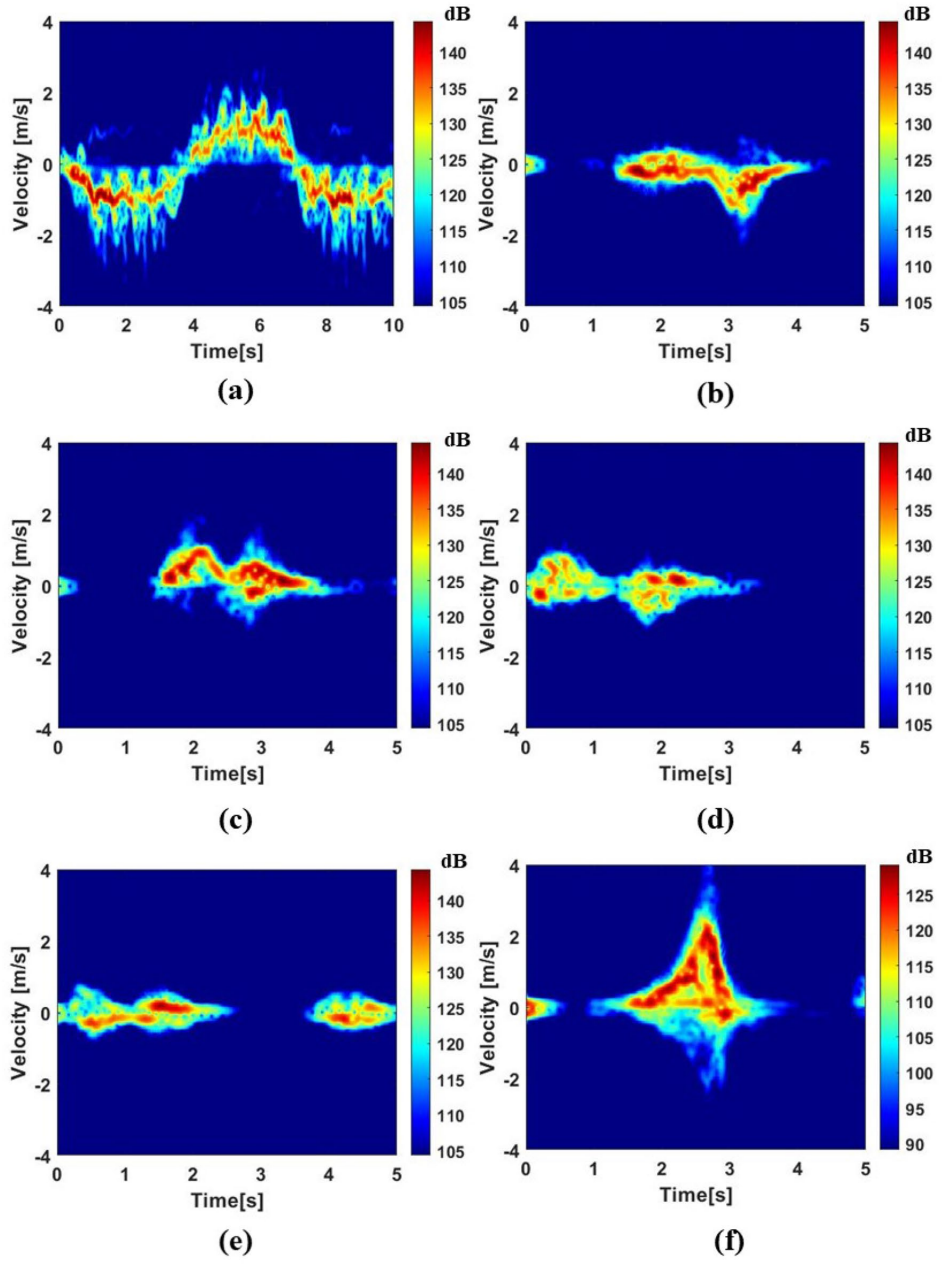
The micro-Doppler signatures of typical samples of the dataset. (a–f) represent activities A1 ~ A6 micro-Doppler spectrogram.
